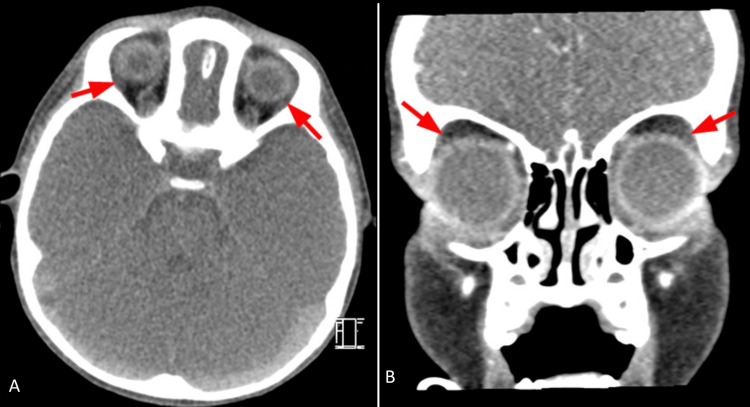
Congenital alacrima An 18-month-old patient presenting with congenital alacrima. Contrast-enhanced computed tomography (CT) images were obtained. Axial (A) and coronal (B) reformat images at the level of the lacrimal fossa show absence of the bilateral lacrimal glands (red arrows).
